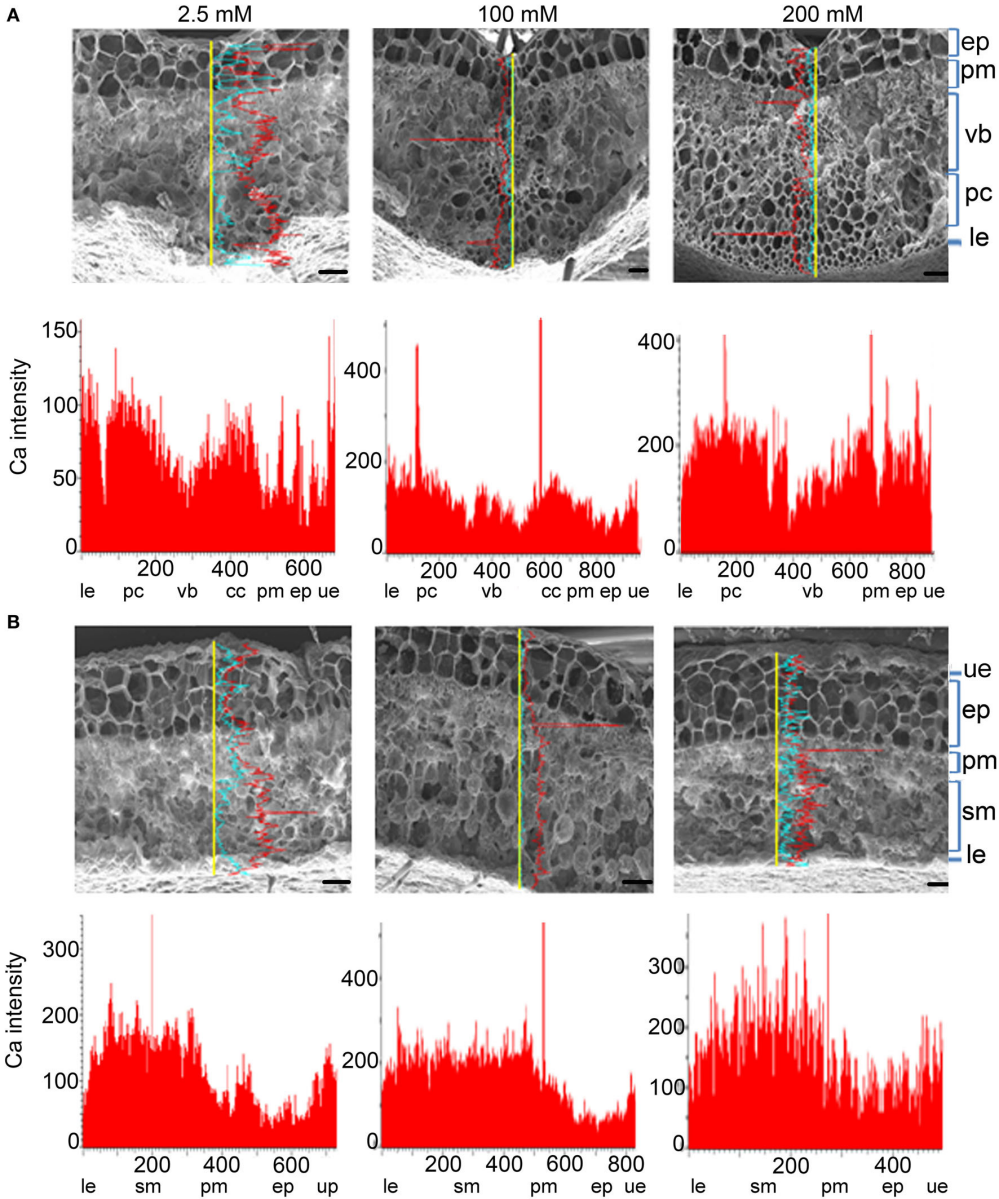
Ca accumulation and distribution in the vein and non-vein parts of *L. pauciflorus* leaves. (A) Vein. (B) Non-vein. Ca content was detected by SEM X-ray line profiles. Plants were treated with 2.5, 100, 200 mM Ca concentrations respectively for 7 days. Yellow line, the scanning line. Red curve, Ca intensity counts. Blue curve, Mg intensity counts. Y-axis, Ca intensity counts; X-axis, position on line scan (μm). Scale bar = 100 μm. ue, upper epidermis; pm, palisade mesophyll; cc, collenchyme; vb, vascular bundle; pc, parenchyma; ep, epithelial cells; sm, spongy mesophyll; le, lower epidermis.
